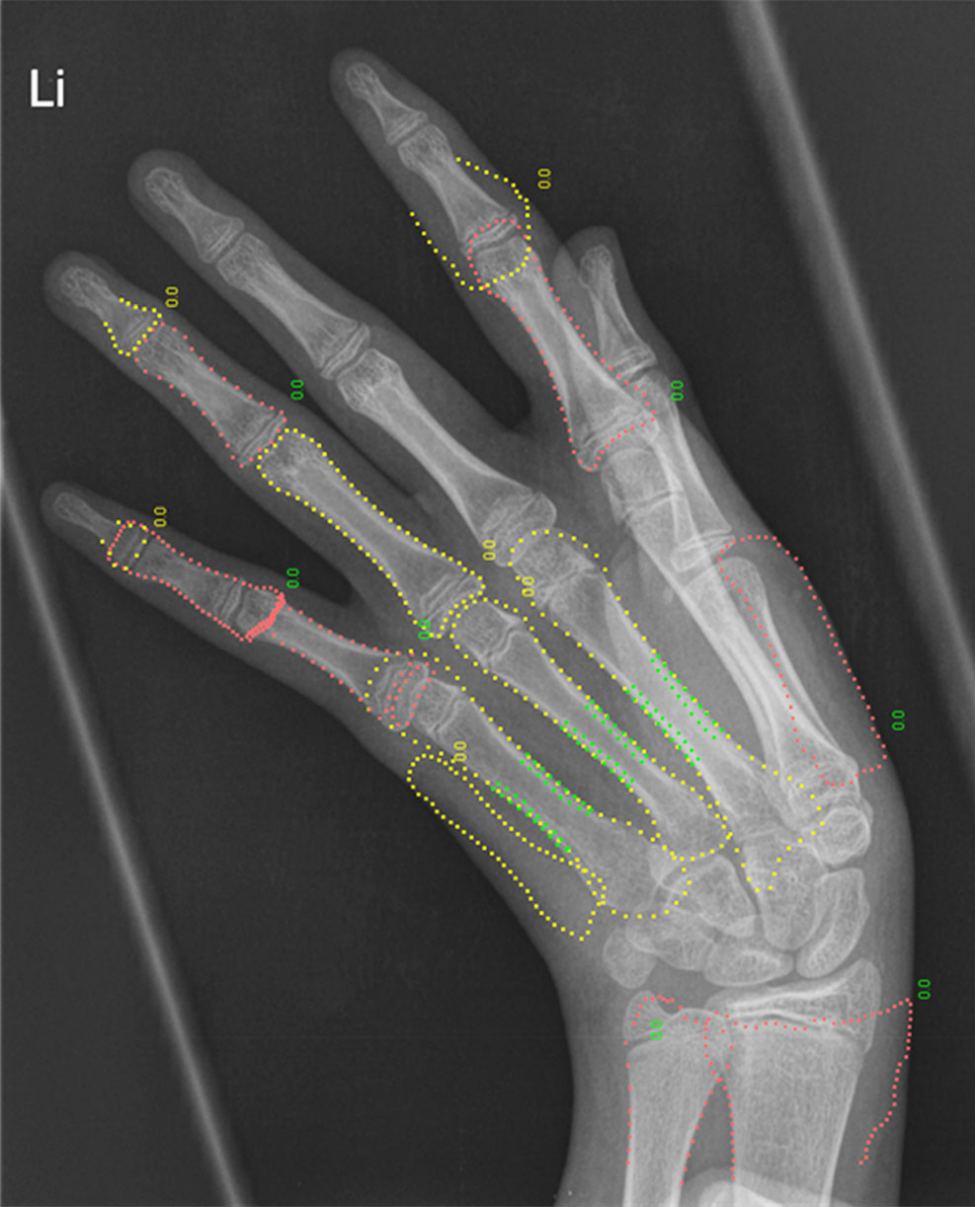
Failed bone age determination using a hand radiograph in a 13-year-old girl with projection of the metacarpals. Hand contractures causing overprojection of the metacarpals prevented BoneXpert software from obtaining the correct positioning of the dotted lines on the bone edges and correct positioning of the regions of interest in this case. *Li* left
